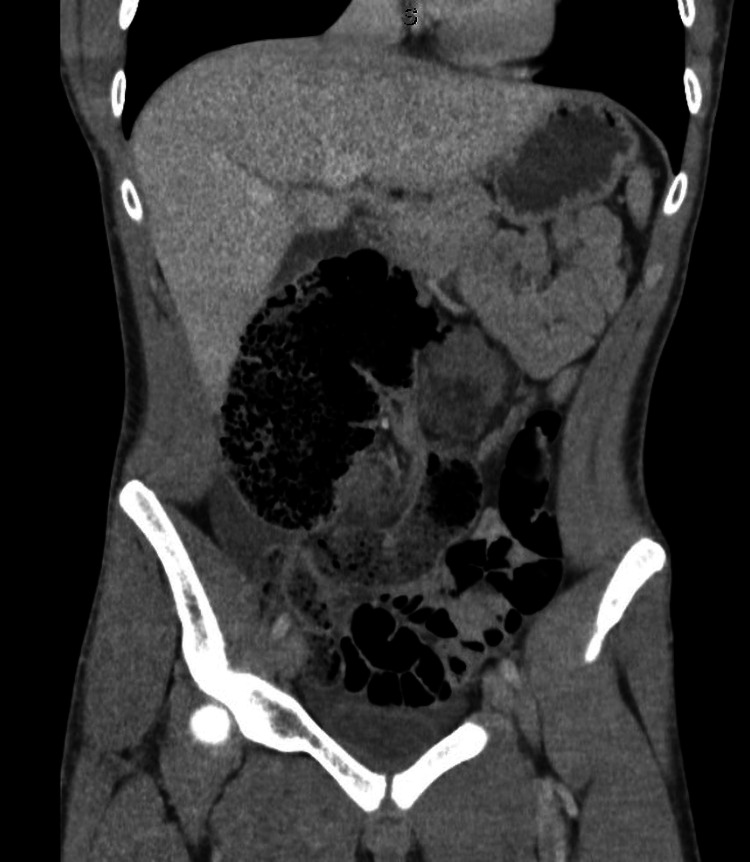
Abdominal CT showing a distended and medially located cecum with fecalization of the distal ileum and decompression of the ascending colon, all concerning for cecal volvulus.
